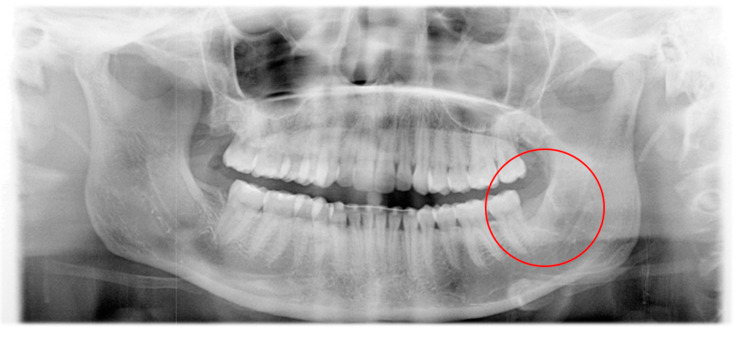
Panoramic radiograph at the first consultation. Alveolus of tooth 48 shows complete healing while ossification is missing in the area of tooth 38.
